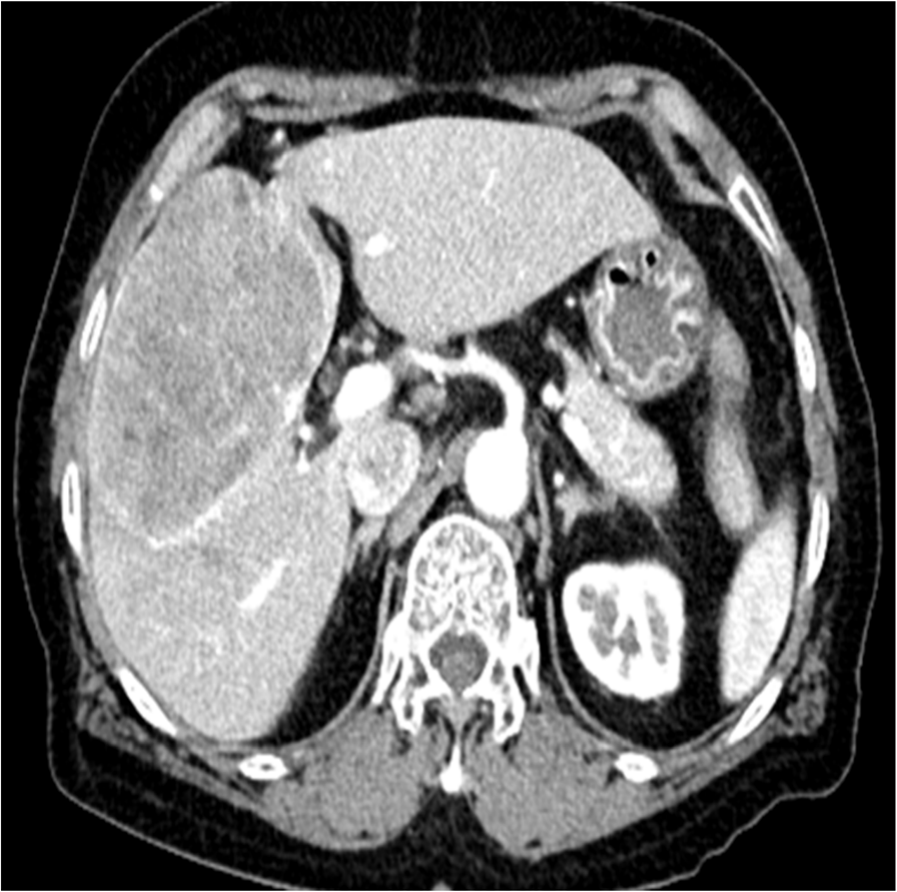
Computed tomography (CT) demonstrating a large tumor (9.5 cm in diameter) in the medial and anterior segments of the liver
